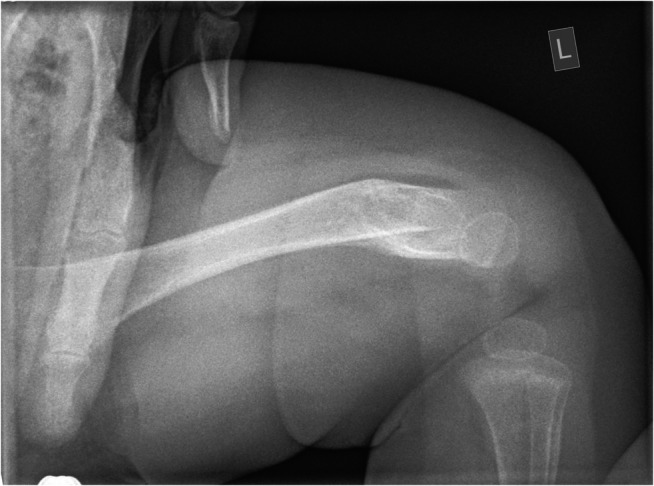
A lateral radiograph of the left femur in a 7-month-old girl shows remodelling
